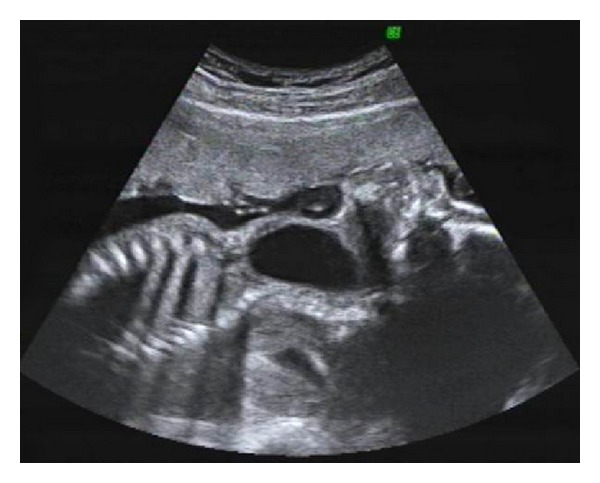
Ultrasound image at 27 weeks' gestation of a bronchogenic cyst.
